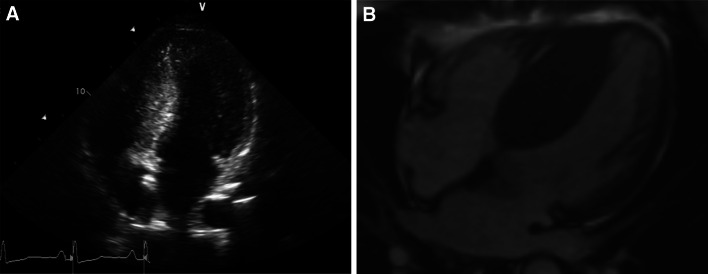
Examples of hypertrophic cardiomyopathy assessed with echocardiography (a) and MRI (b) showing severe septal hypertrophy of the LV
